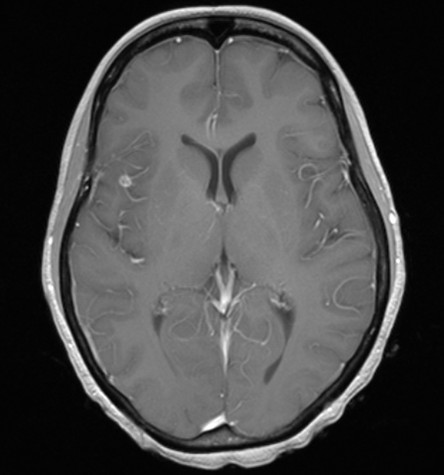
MRI brain scan performed 9 months post chemotherapy. The follow-up scan indicates that the previously noted oedema and the majority of the CNS lesions have resolved.
